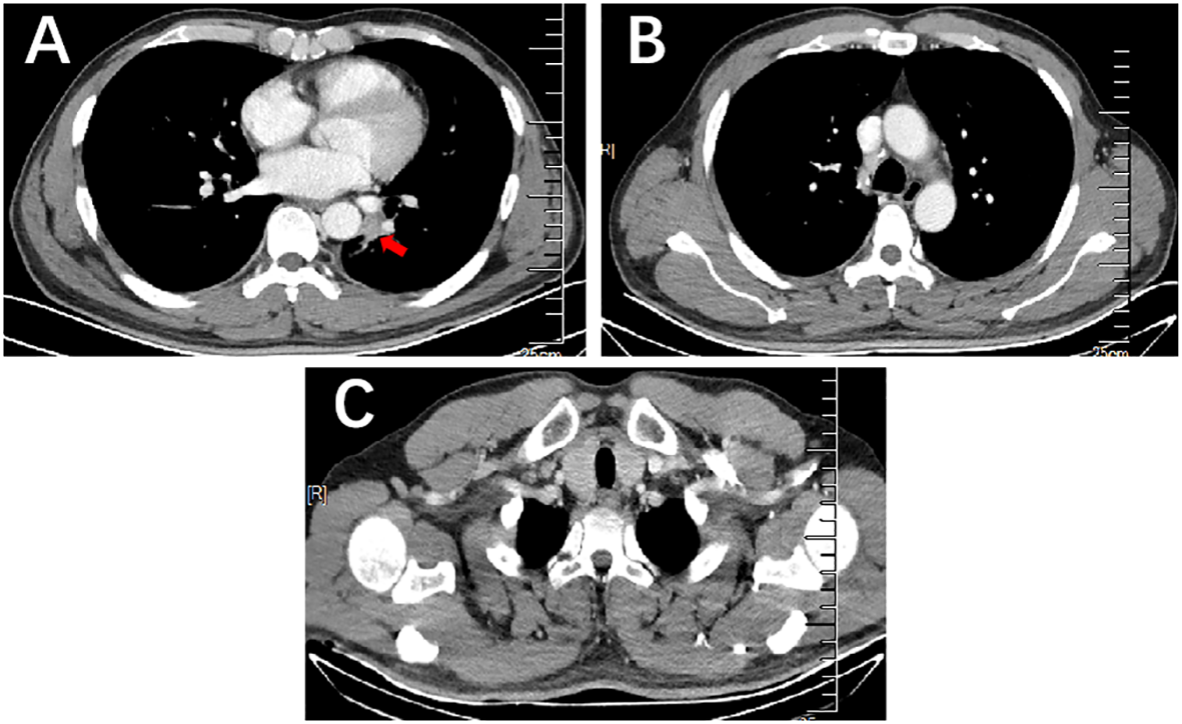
Enhanced CT of the lung mass with metastatic LNs after 2 cycles of immunochemotherapy. (A) Enhanced CT scan showed a decrease in mass size on the left lower lobe compared to that before therapy. (B, C) Enhanced CT scan showed previous metastatic LNs reduced to the standard size and presented normal morphology of reactive lymph nodes in the mediastinum and the left supraclavicular region.
